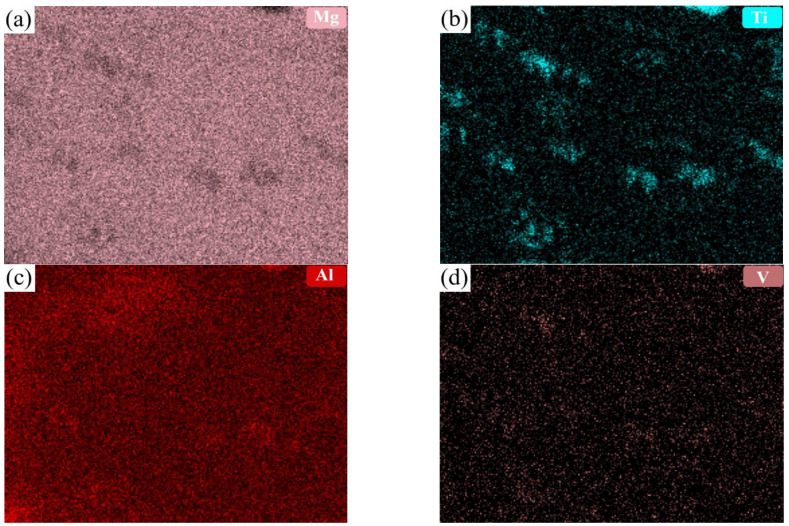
X-ray maps of (a) Mg, (b) Ti, (c) Al, and (d) V in Figure 2b.
